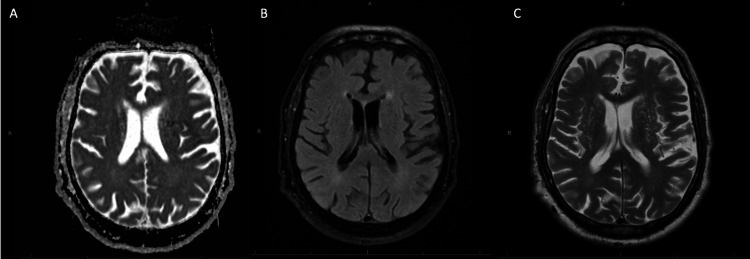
MRI Brain Imaging With and Without Contrast MRI Brain with and without contrast images demonstrating mild, chronic ischemic changes with no evidence of acute or subacute infarction. A: Diffusion Weighted Imaging (DWI), B: T1 weighted sequence, C: T2 fluid attenuated inversion recovery (FLAIR) sequence
